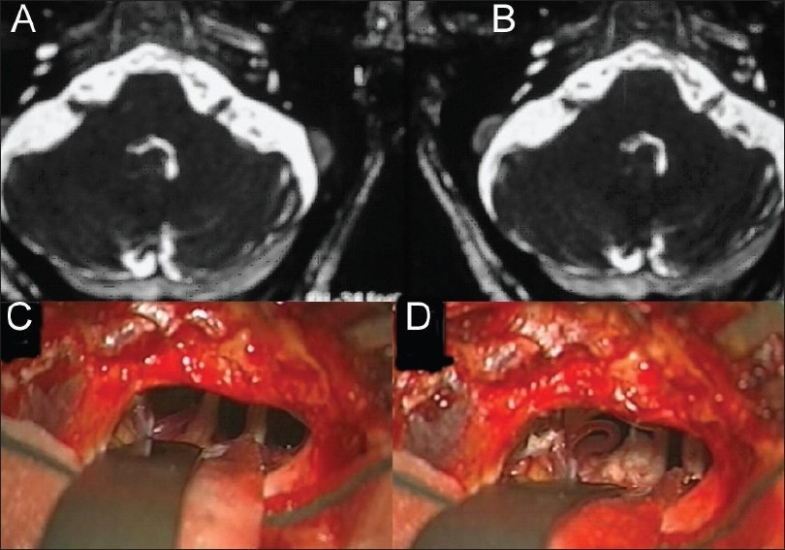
67 yo male patient has a 7 years history of right trigeminal neuralgia and no response to regular medical treatment, above image is T2 weighted MRI that showed no significant changes Apart from high suspension of right vascular loop artifacts around trigeminal nerve entry zone. A and B pictures during surgery of the same patient: picture A there is a compression of the right trigeminal nerve by superior cerebellar artery, picture B showed separation of the artery and nerve with a piece of Teflon
